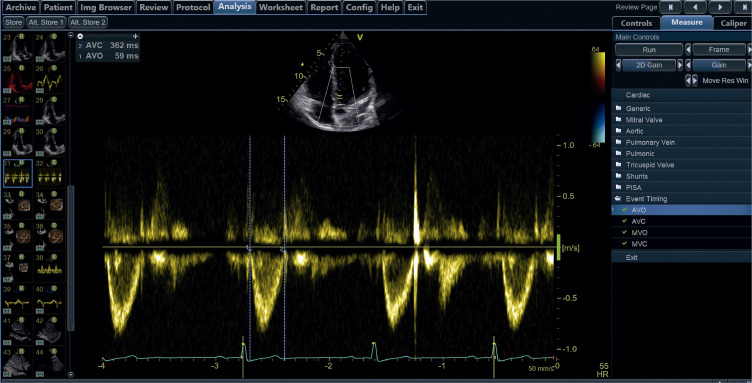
Marcação de eventos cardíacos com o uso do Doppler espectral. Em A, observamos a marcação de abertura (MVO) e o fechamento da valva mitral (MVC) através do influxo mitral. Em B, observamos a marcação de abertura (AVO) e o fechamento da valva aórtica (AVC) através do fluxo da via de saída do ventrículo esquerdo.
